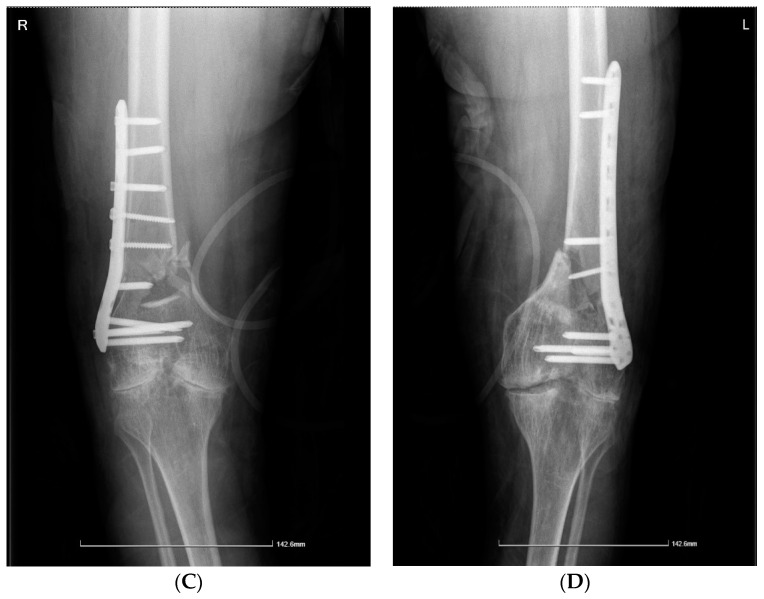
(A,B) Case 2—Computed tomography image: bilateral femoral supracondylar comminuted fracture. (C,D) Case 2—X-ray of right and left femur and knee. Open post-reduction status and internal fixation with present osteosynthesis material (plate with screws) at the level of the diaphysis and distal femoral epiphysis, following a femoral supracondylar comminuted fracture.
